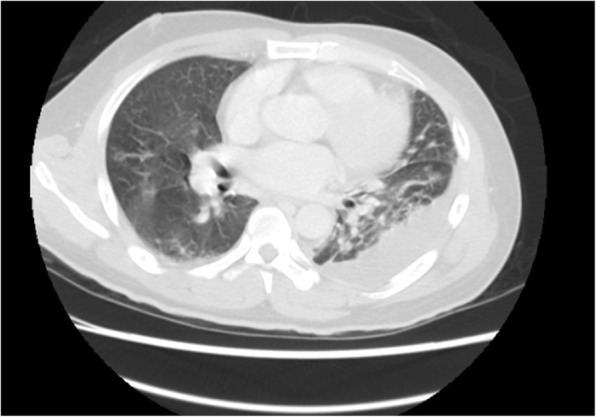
Chest CT shows loculated pleural effusion, consolidations with central lucency collection in both lungs, and mild pericardial effusion
